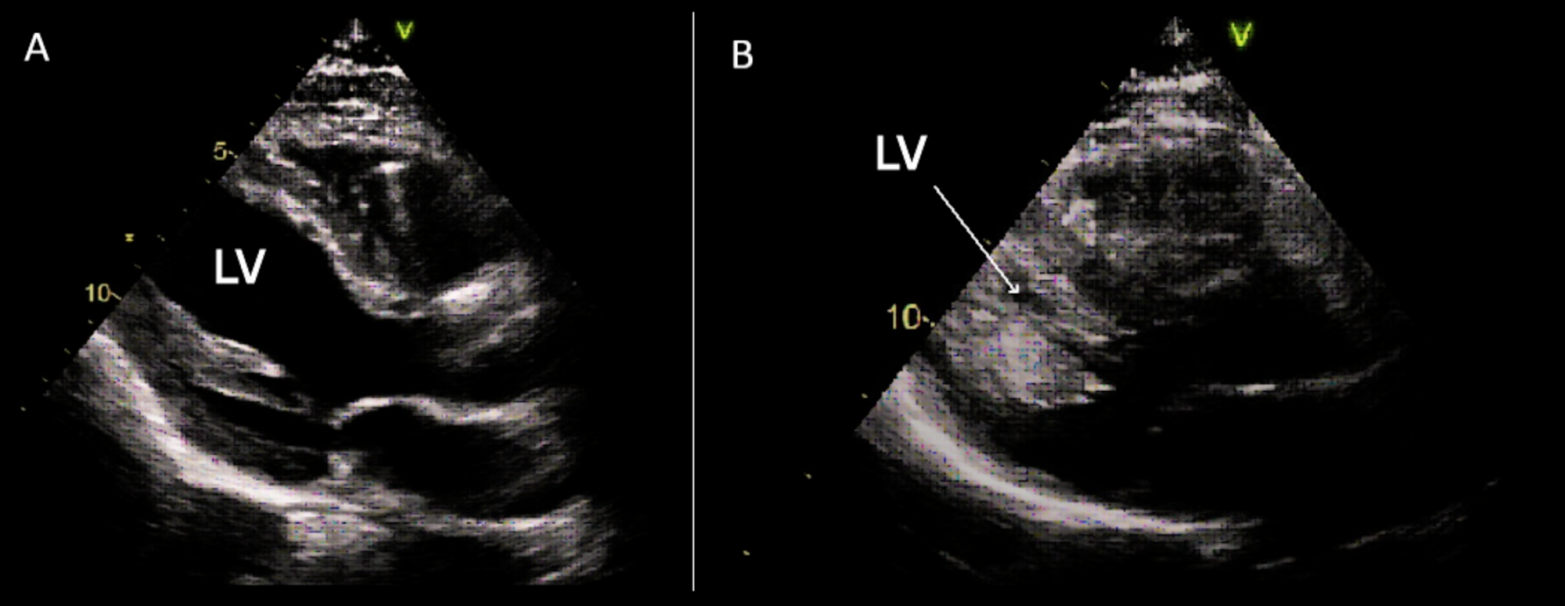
A) Transthoracic echocardiogram performed on day one of hospitalization showing an ejection fraction (EF) of 10%. B) Transthoracic echocardiogram performed on day six of hospitalization showing an EF of 61% LV: Left ventricle
